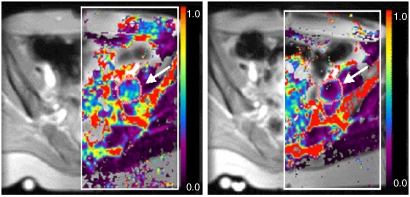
Example of *K*^trans^ maps, superimposed on T1-weighted images of a patient with primary peritoneal carcinoma with left pelvic side wall nodal metastases (arrow). Images are before and 4 h after the first dose of Combretastatin (52 mg m^−2^). The colour scale ranges from *K*^trans^ values of 0 to 1 min^−1^. A dramatic reduction of transfer constant is seen. This was published with kind permission from the Journal of Magnetic Resonance Imaging (Padhani, 2002).
